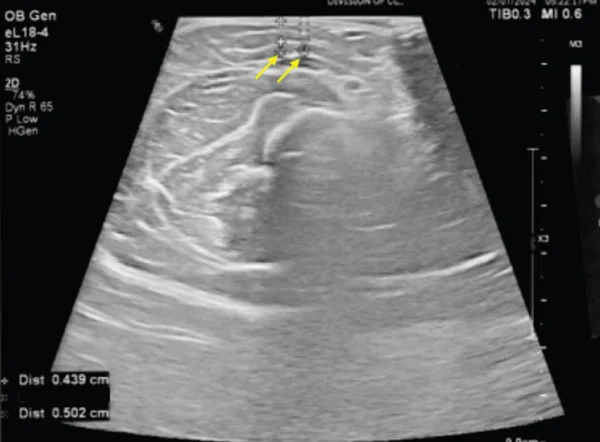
Ultrasound image obtained during evaluation of the upper left extremity. A high‐frequency transducer was used to confirm the presence of two nonpalpable Nexplanon implants (yellow arrows) located suprafascial above the muscle within subcutaneous tissue.
